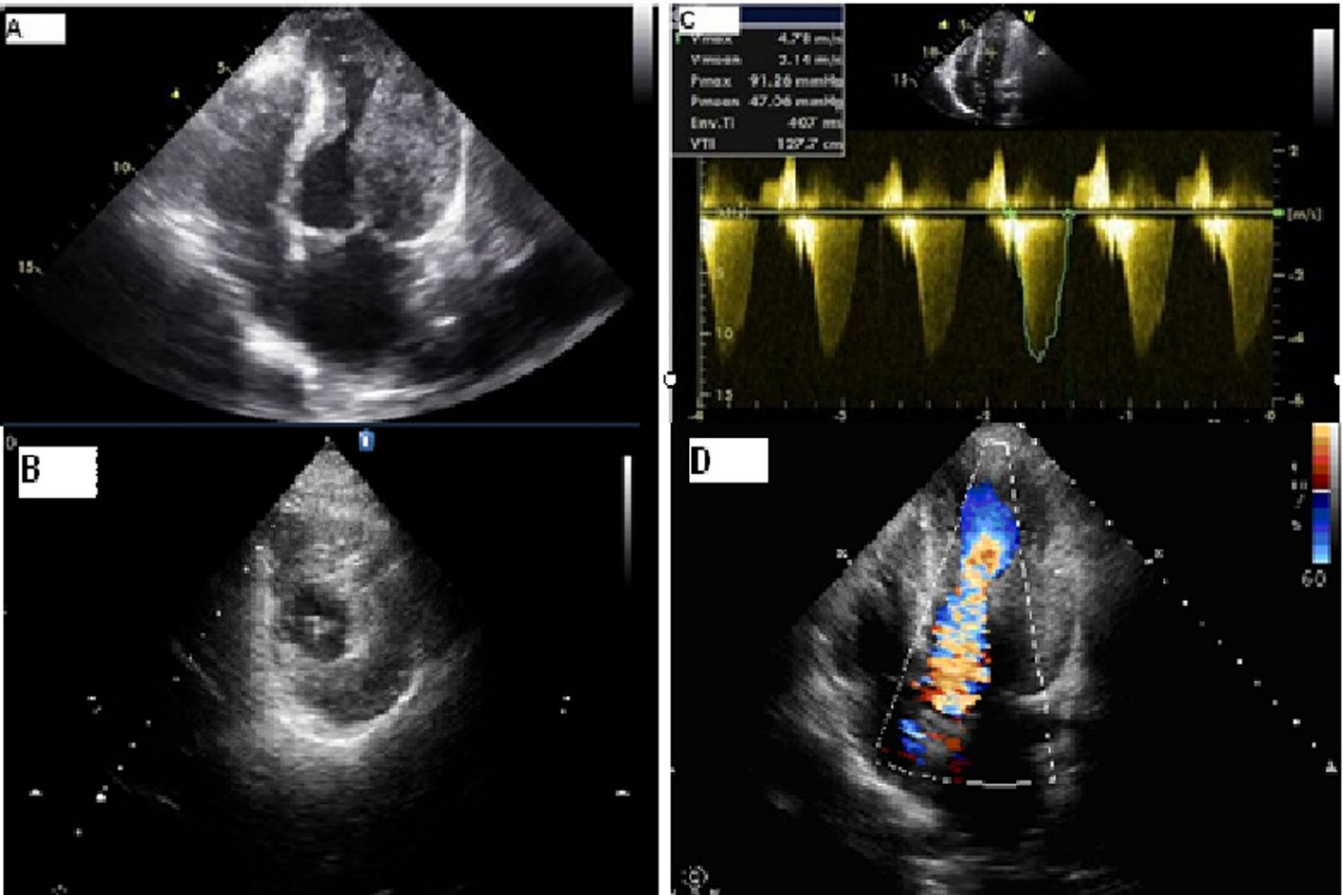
Two-dimensional echocardiogram. Apical four chamber view shows severe wall thickness of the lateral free wall and mid ventricular septum (A). Parasternal short axis view shows severe wall thickness of the posterior and lateral left ventricular free wall, and mid ventricular septum (B). Continuous-wave Doppler study demonstrating increased velocity in the mid ventricular section (C). Color doppler imaging demonstrating flow acceleration due to mid ventricular obstruction (D).
